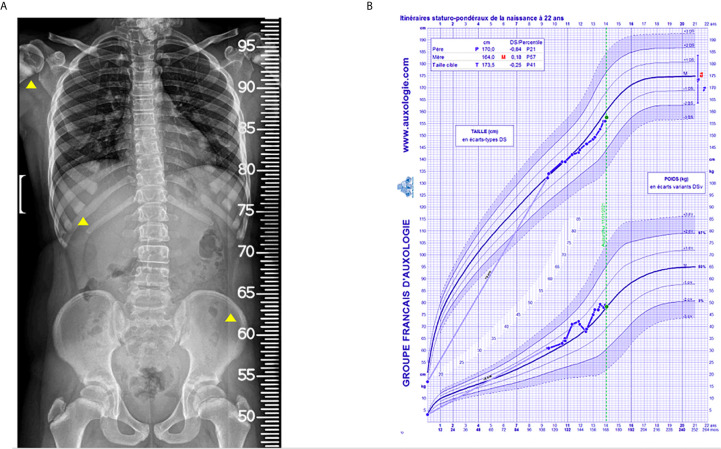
Spine, thoracic and hip radiographic view done by EOS and growth chart. (A) The radiograph was done after 2 years of the 2^nd^ course of denosumab showing sclerotic bands at ribs, hips and shoulders. (B) Evolution of growth.
